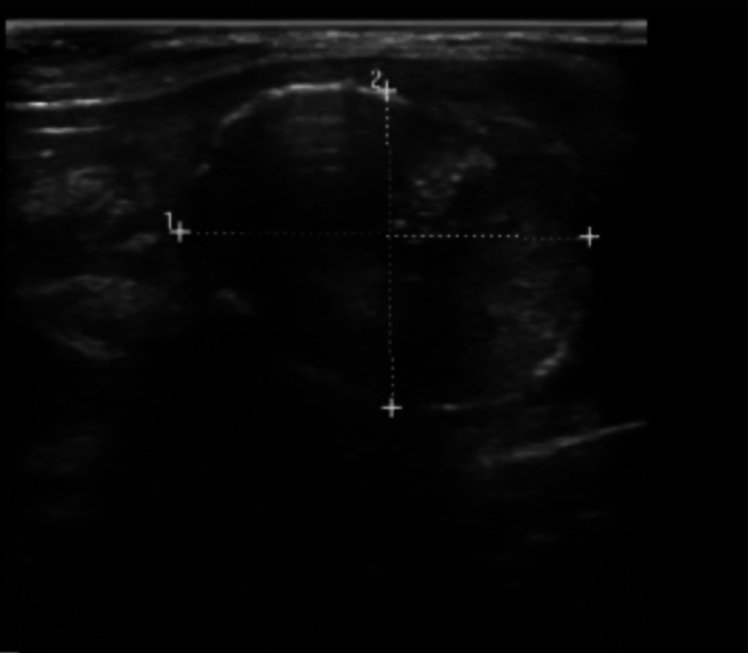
Thyroid nodule on ultrasound
